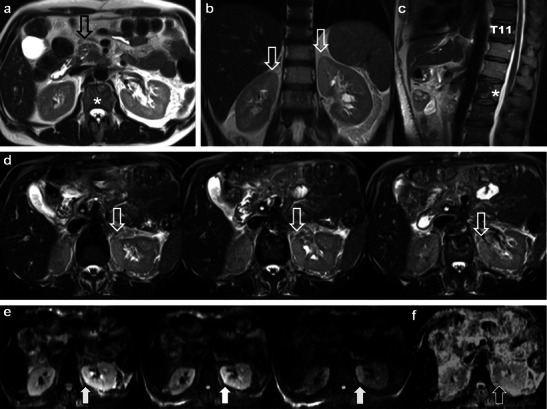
A 50-year-old man with pancreatic head cancer. Baseline axial T2 single-shot fast spin echo (ssFSE) pre-treatment MRI (a) demonstrates the primary tumour (*open black arrow*) and L2 metastasis (*asterisk*). The patient underwent Whipple’s procedure and external beam radiotherapy (RT) for L2 metastasis (30Gy over ten fractions). Follow-up MRI was performed 9 months after treatment. Coronal (b), sagittal (c) T2 ssFSE and axial fat suppressed T2 ssFSE (d) demonstrate increased T2 signal intensity in the medial upper pole renal cortices (*open white arrows* in b and d) consistent with acute radiation nephropathy. Note marrow replacement in the spine and partially treated L2 metastasis (*asterisk*) in c. Areas of radiation nephropathy demonstrate restricted diffusion in the medial upper poles (*solid white arrows*) on axial fat suppressed diffusion weighted echo planar (EPI) images (B50, B400 and B800—*left to right* in e). There is corresponding low signal intensity (*black arrow*) on ADC map (f)
